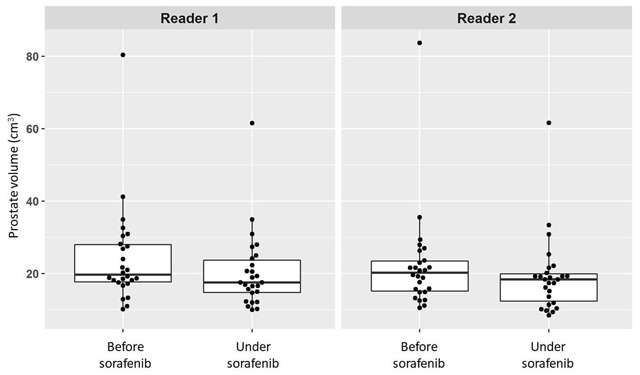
Plotted prostate volumes on the baseline and final follow-up CT as assessed by two readers.
